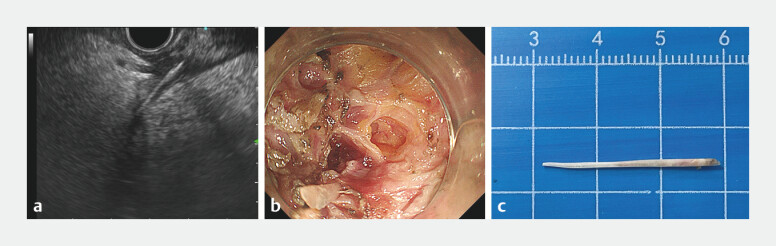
Endoscopic ultrasound (EUS)-guided endoscopic full-thickness resection to remove the foreign body.
